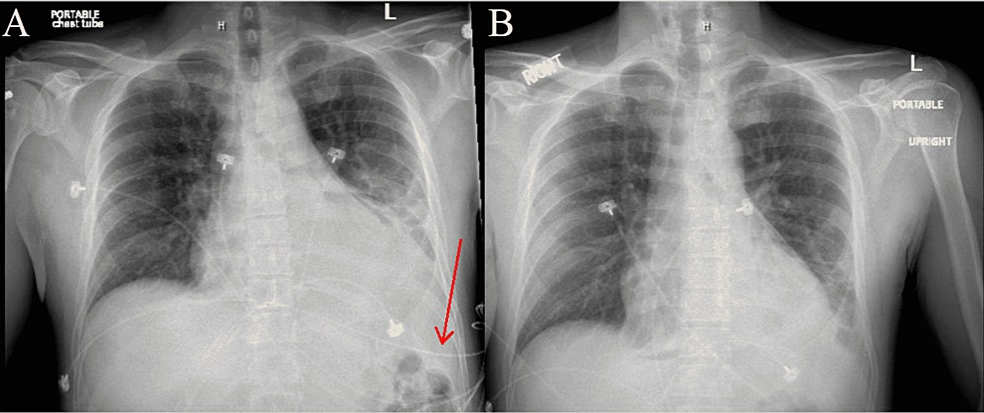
(A) AP chest X-ray on September 2021 prior to pericardial window. Cardiomegaly is present and red arrow points to small left pleural effusion. (B) AP chest X-ray on September 2021 after pericardial window. Cardiomegaly is improved and size of cardiac silhouette is reduced.
